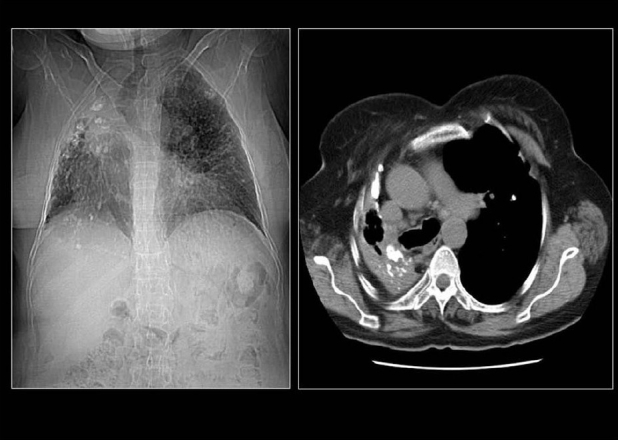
A chest radiograph and CT showing features of old healed TB. Note the loss of lung volume/fibrosis in the right upper zone and the associated pleural calcification due to a previous tuberculous empyema. Calcific granulomas are also noted in the left apical region
